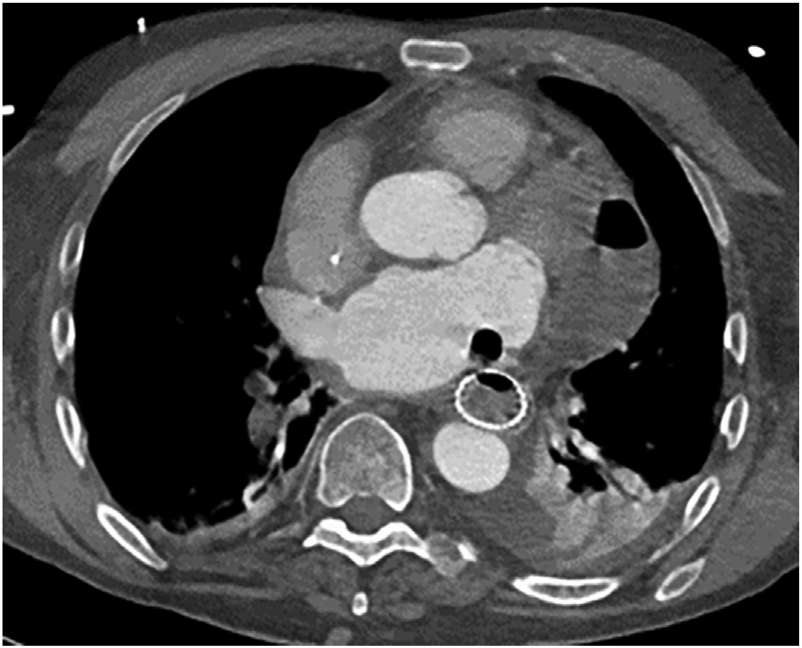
Cardiac computed tomography angiograph demonstrating progression to an atrial esophageal fistula with a complex fluid collection within the pericardium.
